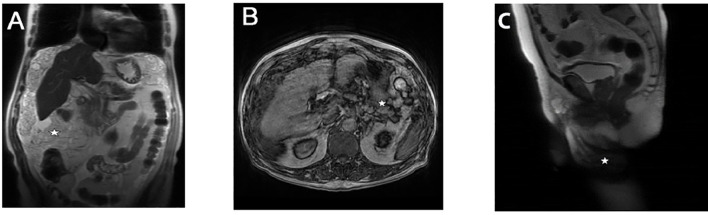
Preoperative MRI of the patient. (A) MRI shows multiple patchy low T1WI and high T2WI signals in the right scrotal area, with no high signal on DWI, and displaceent of both testes due to compression. (B) MRI indicates the presence of hydrocele in the left testis. (C) MRI reveals slightly enlarged lymph nodes in both inguinal regions, with no detectable tumors in the pelvic and abdominal cavities.
